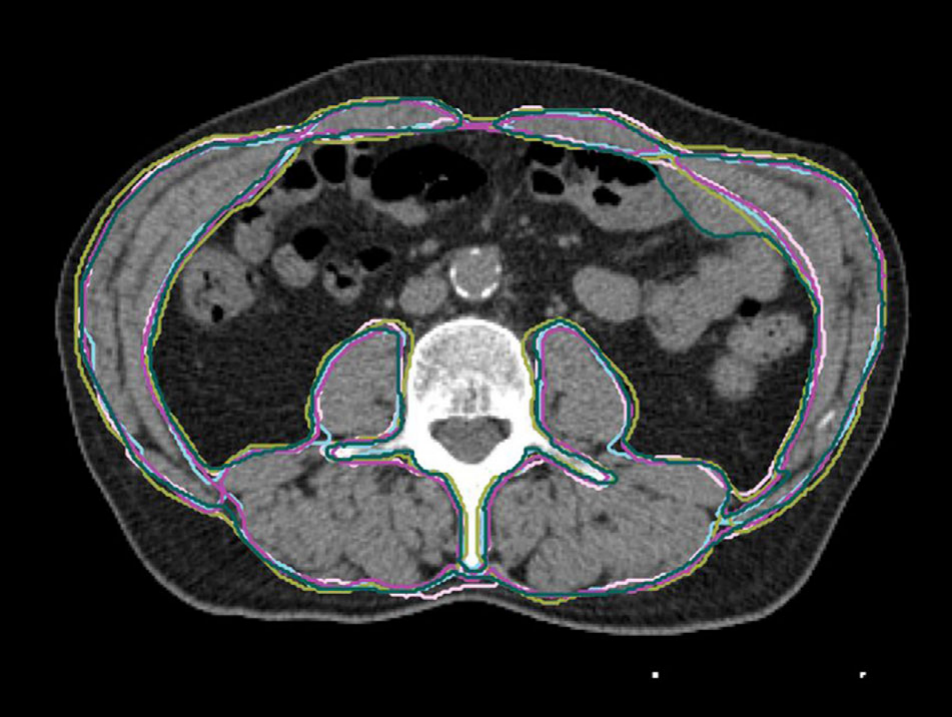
An example CT slice from our inter‐observer study, with multiple observer delineations in different colors
